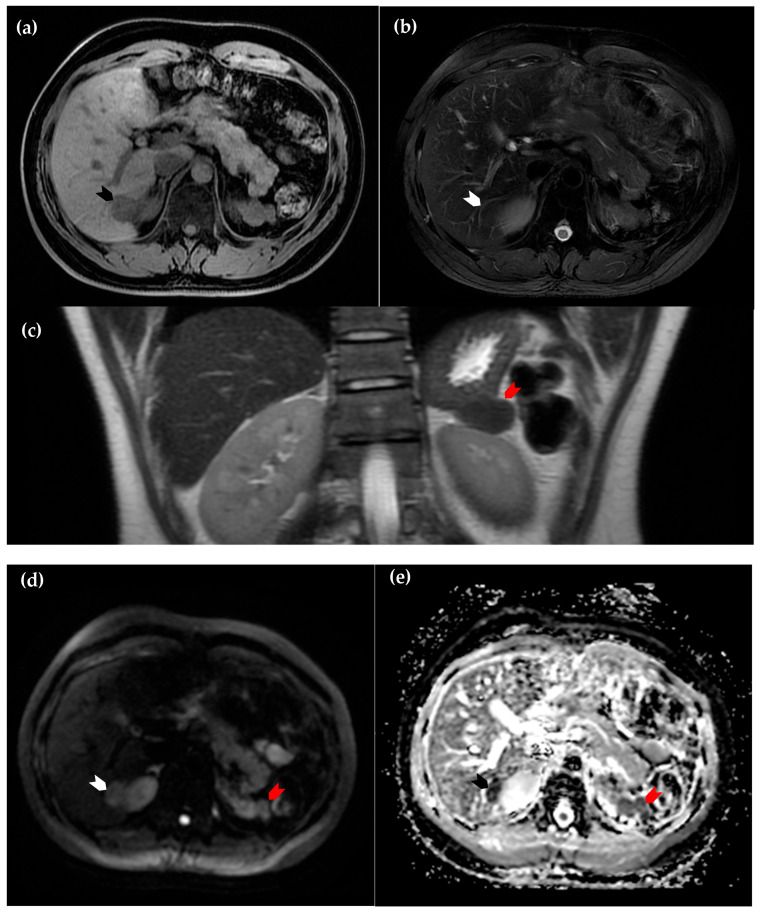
Magnetic resonance imaging of the liver. (a) Axial view of the T1 sequence, in which the intrahepatic lesion is hypointense in comparison to surrounding normal hepatic parenchyma (black arrowhead); (b) axial view of the T2 sequence, in which the lesion is nearly isointense (white arrowhead); (c) coronal view of the T2 sequence. The suspected perinephric splenosis (red arrowhead) again exhibits similar attenuation to the intrahepatic lesion (white arrowhead in (d) and black arrowhead in (e)), as well as characteristics of restricted diffusion on diffusion-weighted imaging (d) and the corresponding apparent diffusion coefficient map (e). These findings support the diagnosis of intrahepatic splenosis [2].
